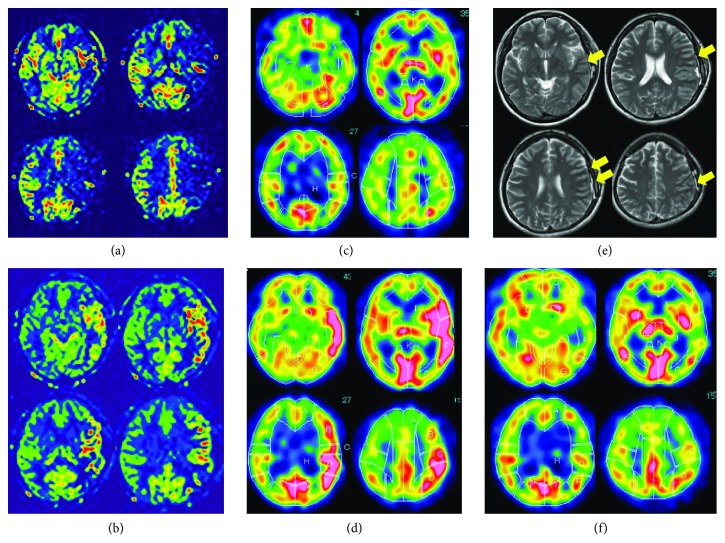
Pre- and postoperative neuroradiologic examinations. (a) Arterial spin labelling (ASL) of MRI showing decrease of CBF in the left brain when the patient presented with right hand weakness and numbness; (b) ASL on postoperative day (POD) 5 showing an increase of CBF in the left cerebrum; (c) preoperative single-photon emission computed tomography (SPECT) showing no apparent laterality in CBF. (d) SPECT on POD12 showing remarkable increase of CBF in the left cerebrum; (e) MRI T2WI on POD16 showing slightly hypointense change in the subcortex of the left cerebrum (arrows). (f) SPECT on POD33 showing normalization of CBF.
